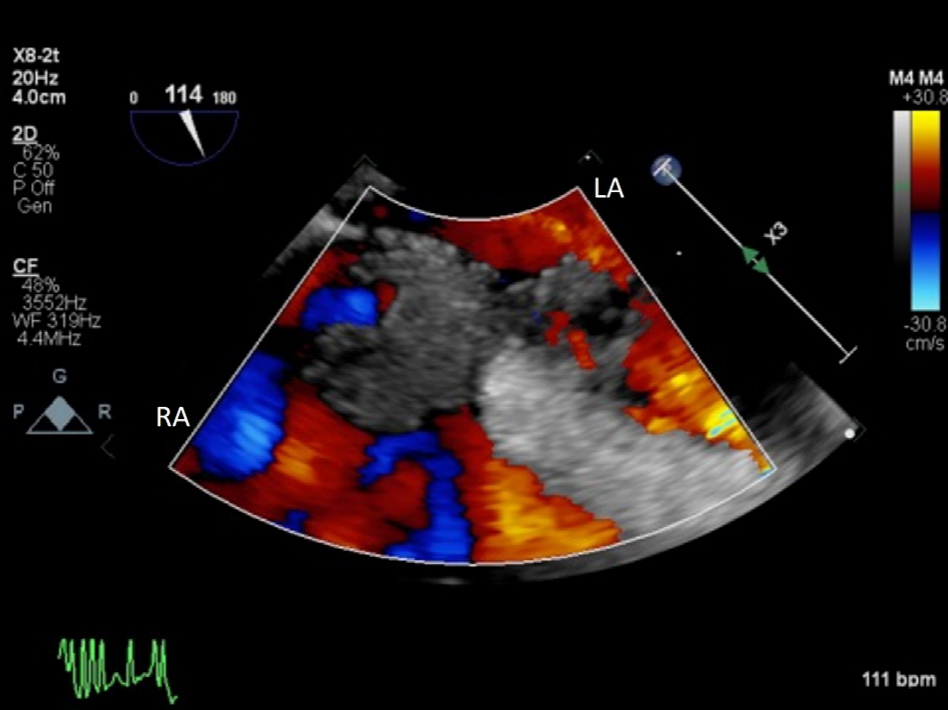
Two-dimensional TEE, midesophageal bicaval view (114°) with color Doppler (using a reduced Nyquist limit) demonstrates absence of flow across the PFO suggesting an occlusive thrombus.
